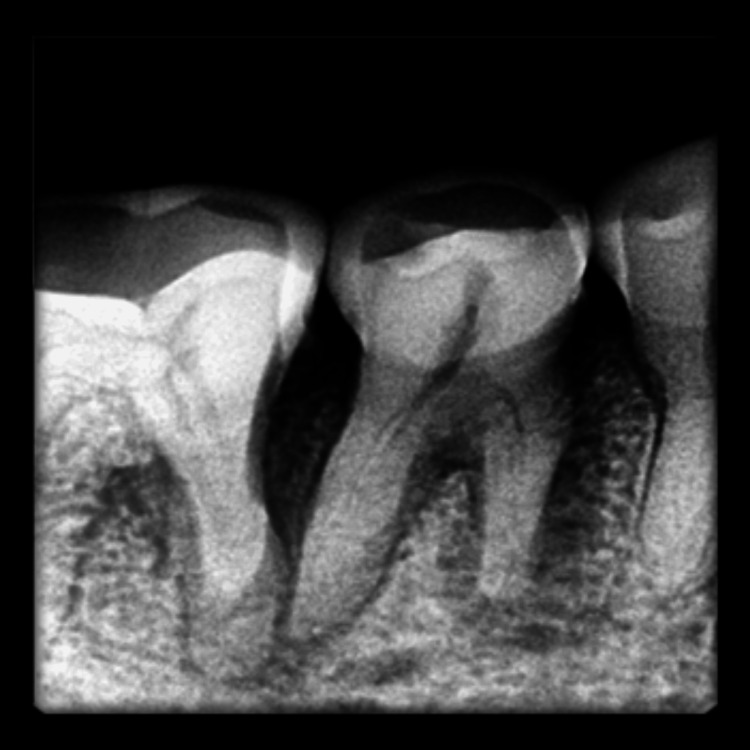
Pre-operative intraoral periapical radiograph of case 1
